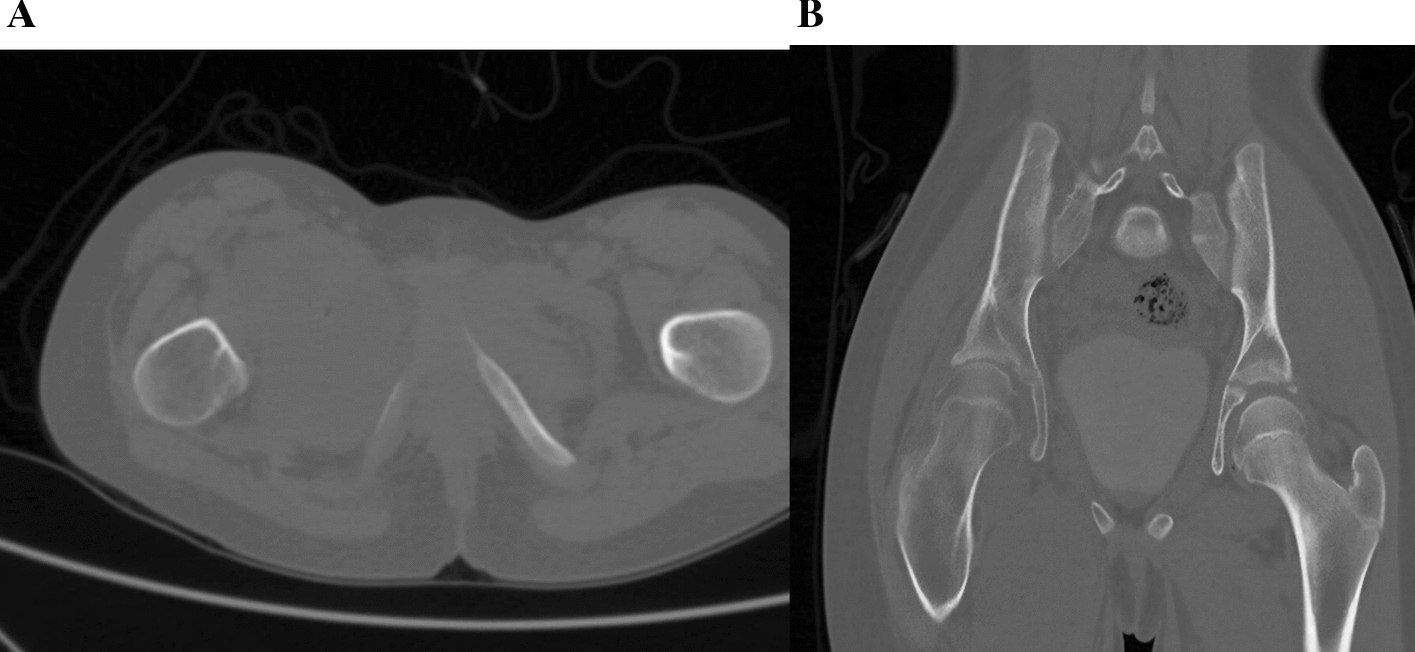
A Axial computed tomography image of the pelvis demonstrating a well-defined hypodense fluid collection located in the adductor muscles, suggestive of an abscess. The collection appears to displace adjacent soft tissue structures without evidence of gas formation or bony involvement. No signs of intrapelvic extension are observed. B Corneal computed tomography image of the pelvis bone window demonstrates significant changes in the right femoral head. There is cortical thinning and irregularity with loss of the normal spherical contour of the femoral head. Mild sclerosis is noted in the femoral neck. The adjacent acetabulum shows evidence of erosion with complete loss of the joint space
